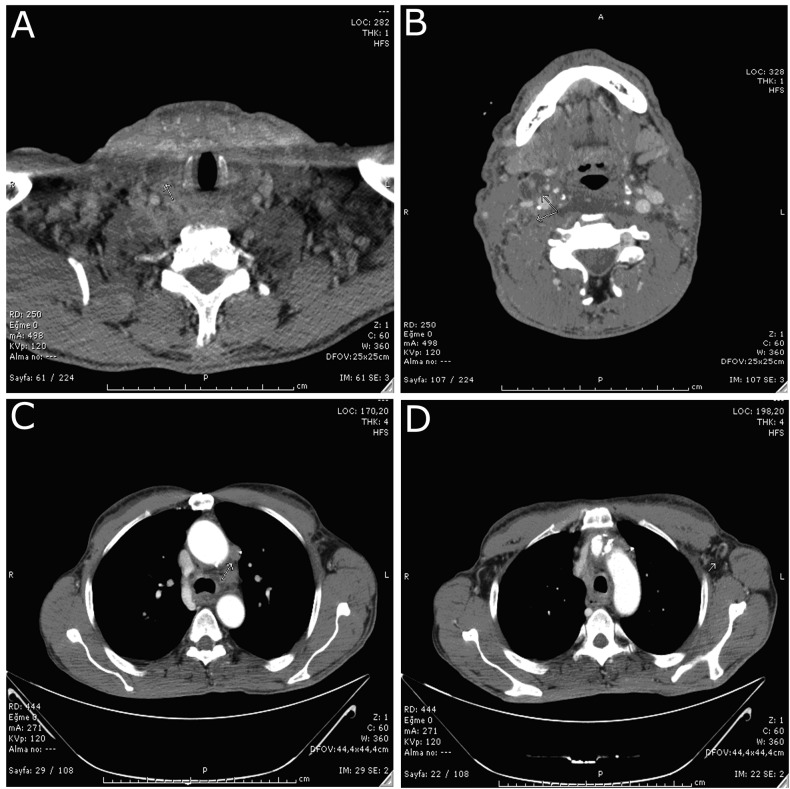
CT scan of the neck revealing a hypodense lesion in the (A) thyroid gland and (B) metastatic cervical lymphadenopathies. CT scan of the thorax showing a metastatic lymphadenopathy in the (C) aorticopulmoner window and (D) lymphadenopathy in the left axilla. CT, computed tomography.
